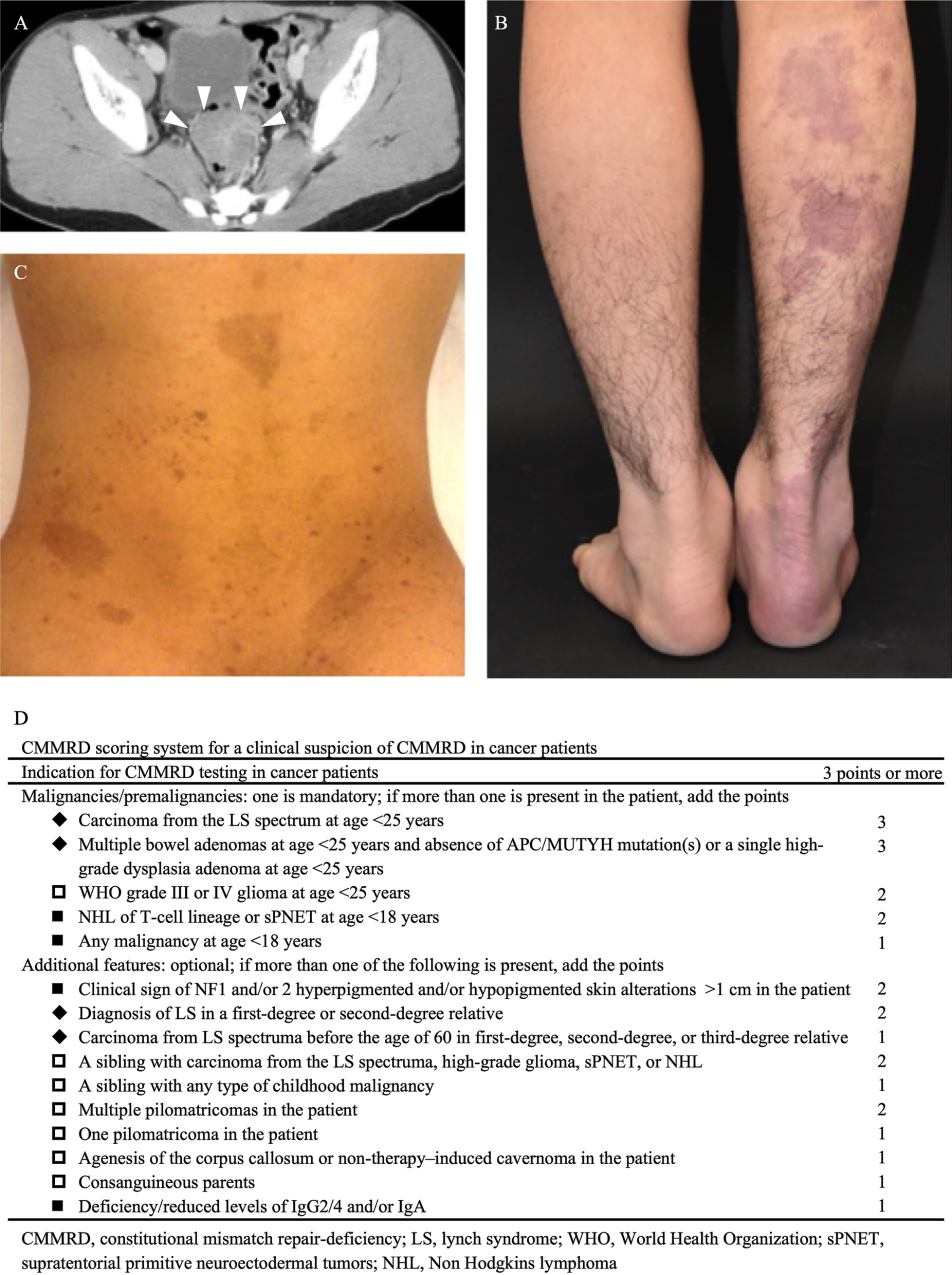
Findings of computed tomography scan, physical examination, and CMMRD scoring system. A Abdominal computed tomography image showing rectal wall thickening without lymphadenopathy (white arrowheads). B, C On physical examination, several café au lait macules and multiple cutaneous hemangiomas on back and right leg, respectively, are visible. D The patient had scores of 5 and 14 points, before and after genetic testing, respectively, according to the CMMRD scoring system. ■, criteria fulfilled before genetic testing; ◆, criteria fulfilled after genetic testing; □, unfulfilled criteria; CMMRD, constitutional mismatch repair deficiency
